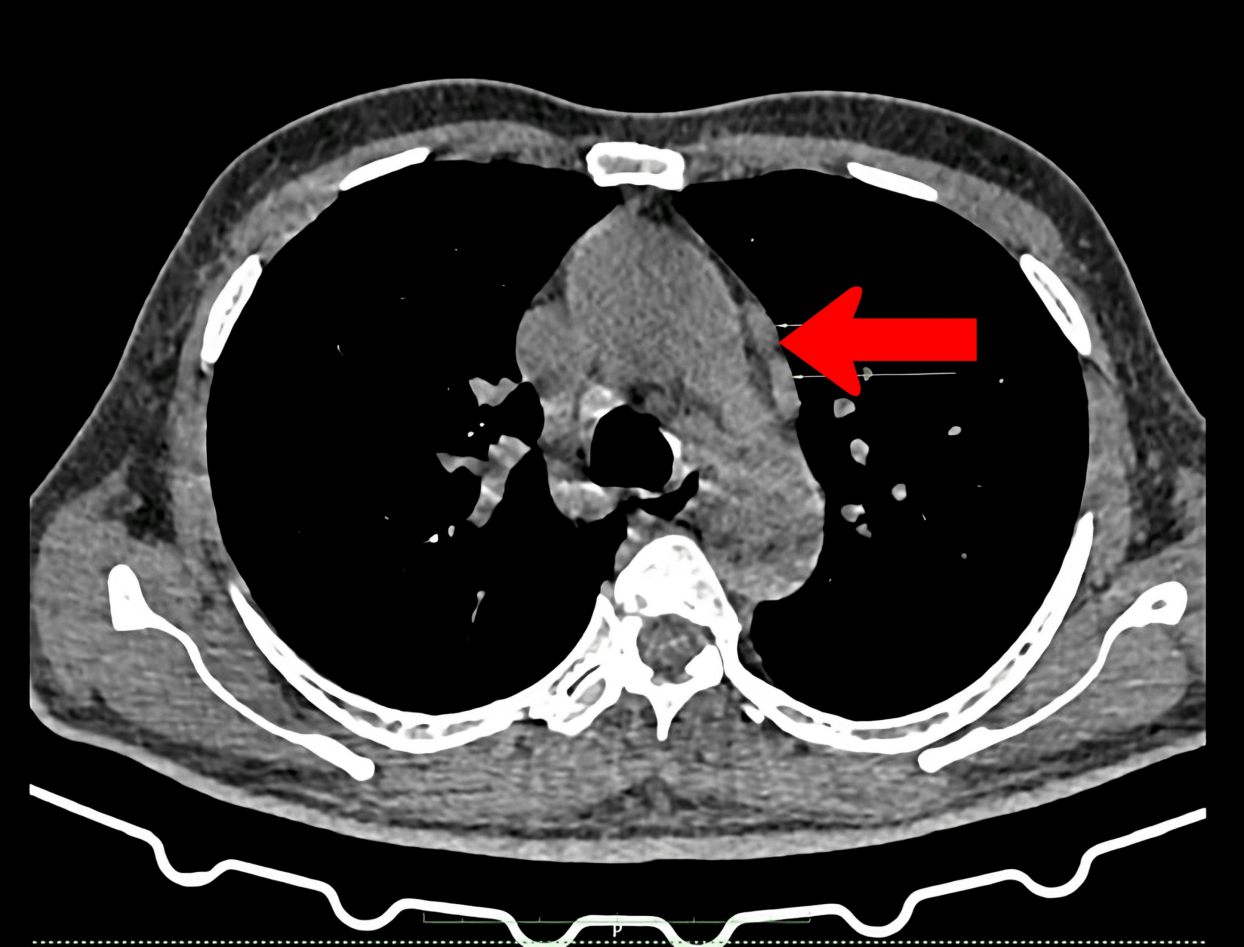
High-resolution CT images of the axial section of the thorax Red arrow points towards the largest mediastinal lymph nodes in the para-aortic window.
